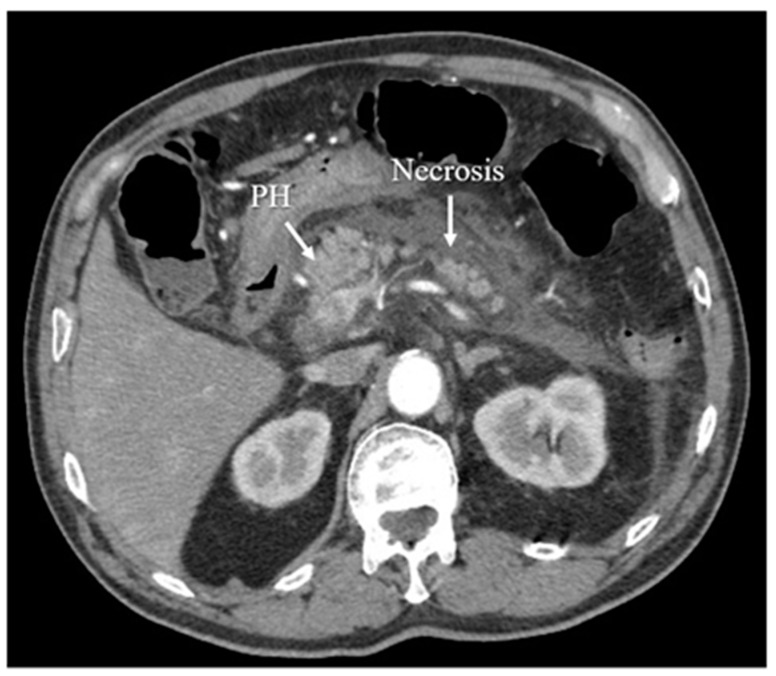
Acute necrotizing pancreatitis in the arterial phase of CT.
